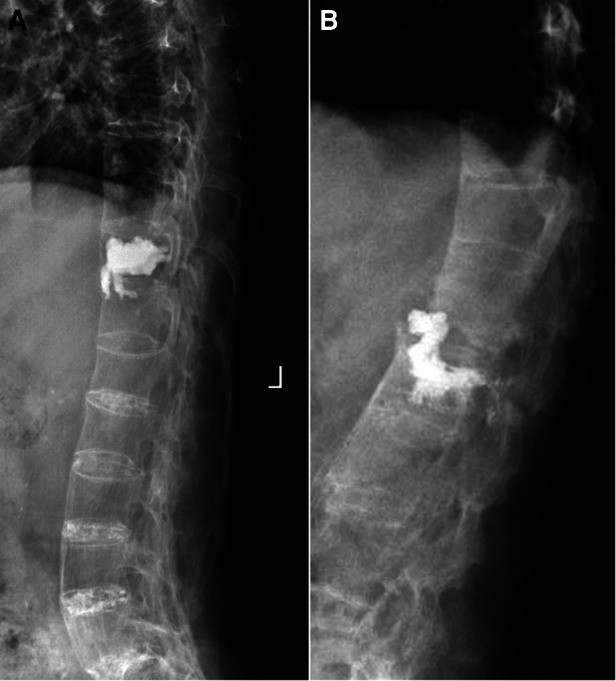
Asymptomatic intradiscal polymethylmethacrylate cement leakage was found in two patients by postoperative x-ray. The cement respectively leaked into the lower (A) and upper (B) discs of the fractured vertebrae.
